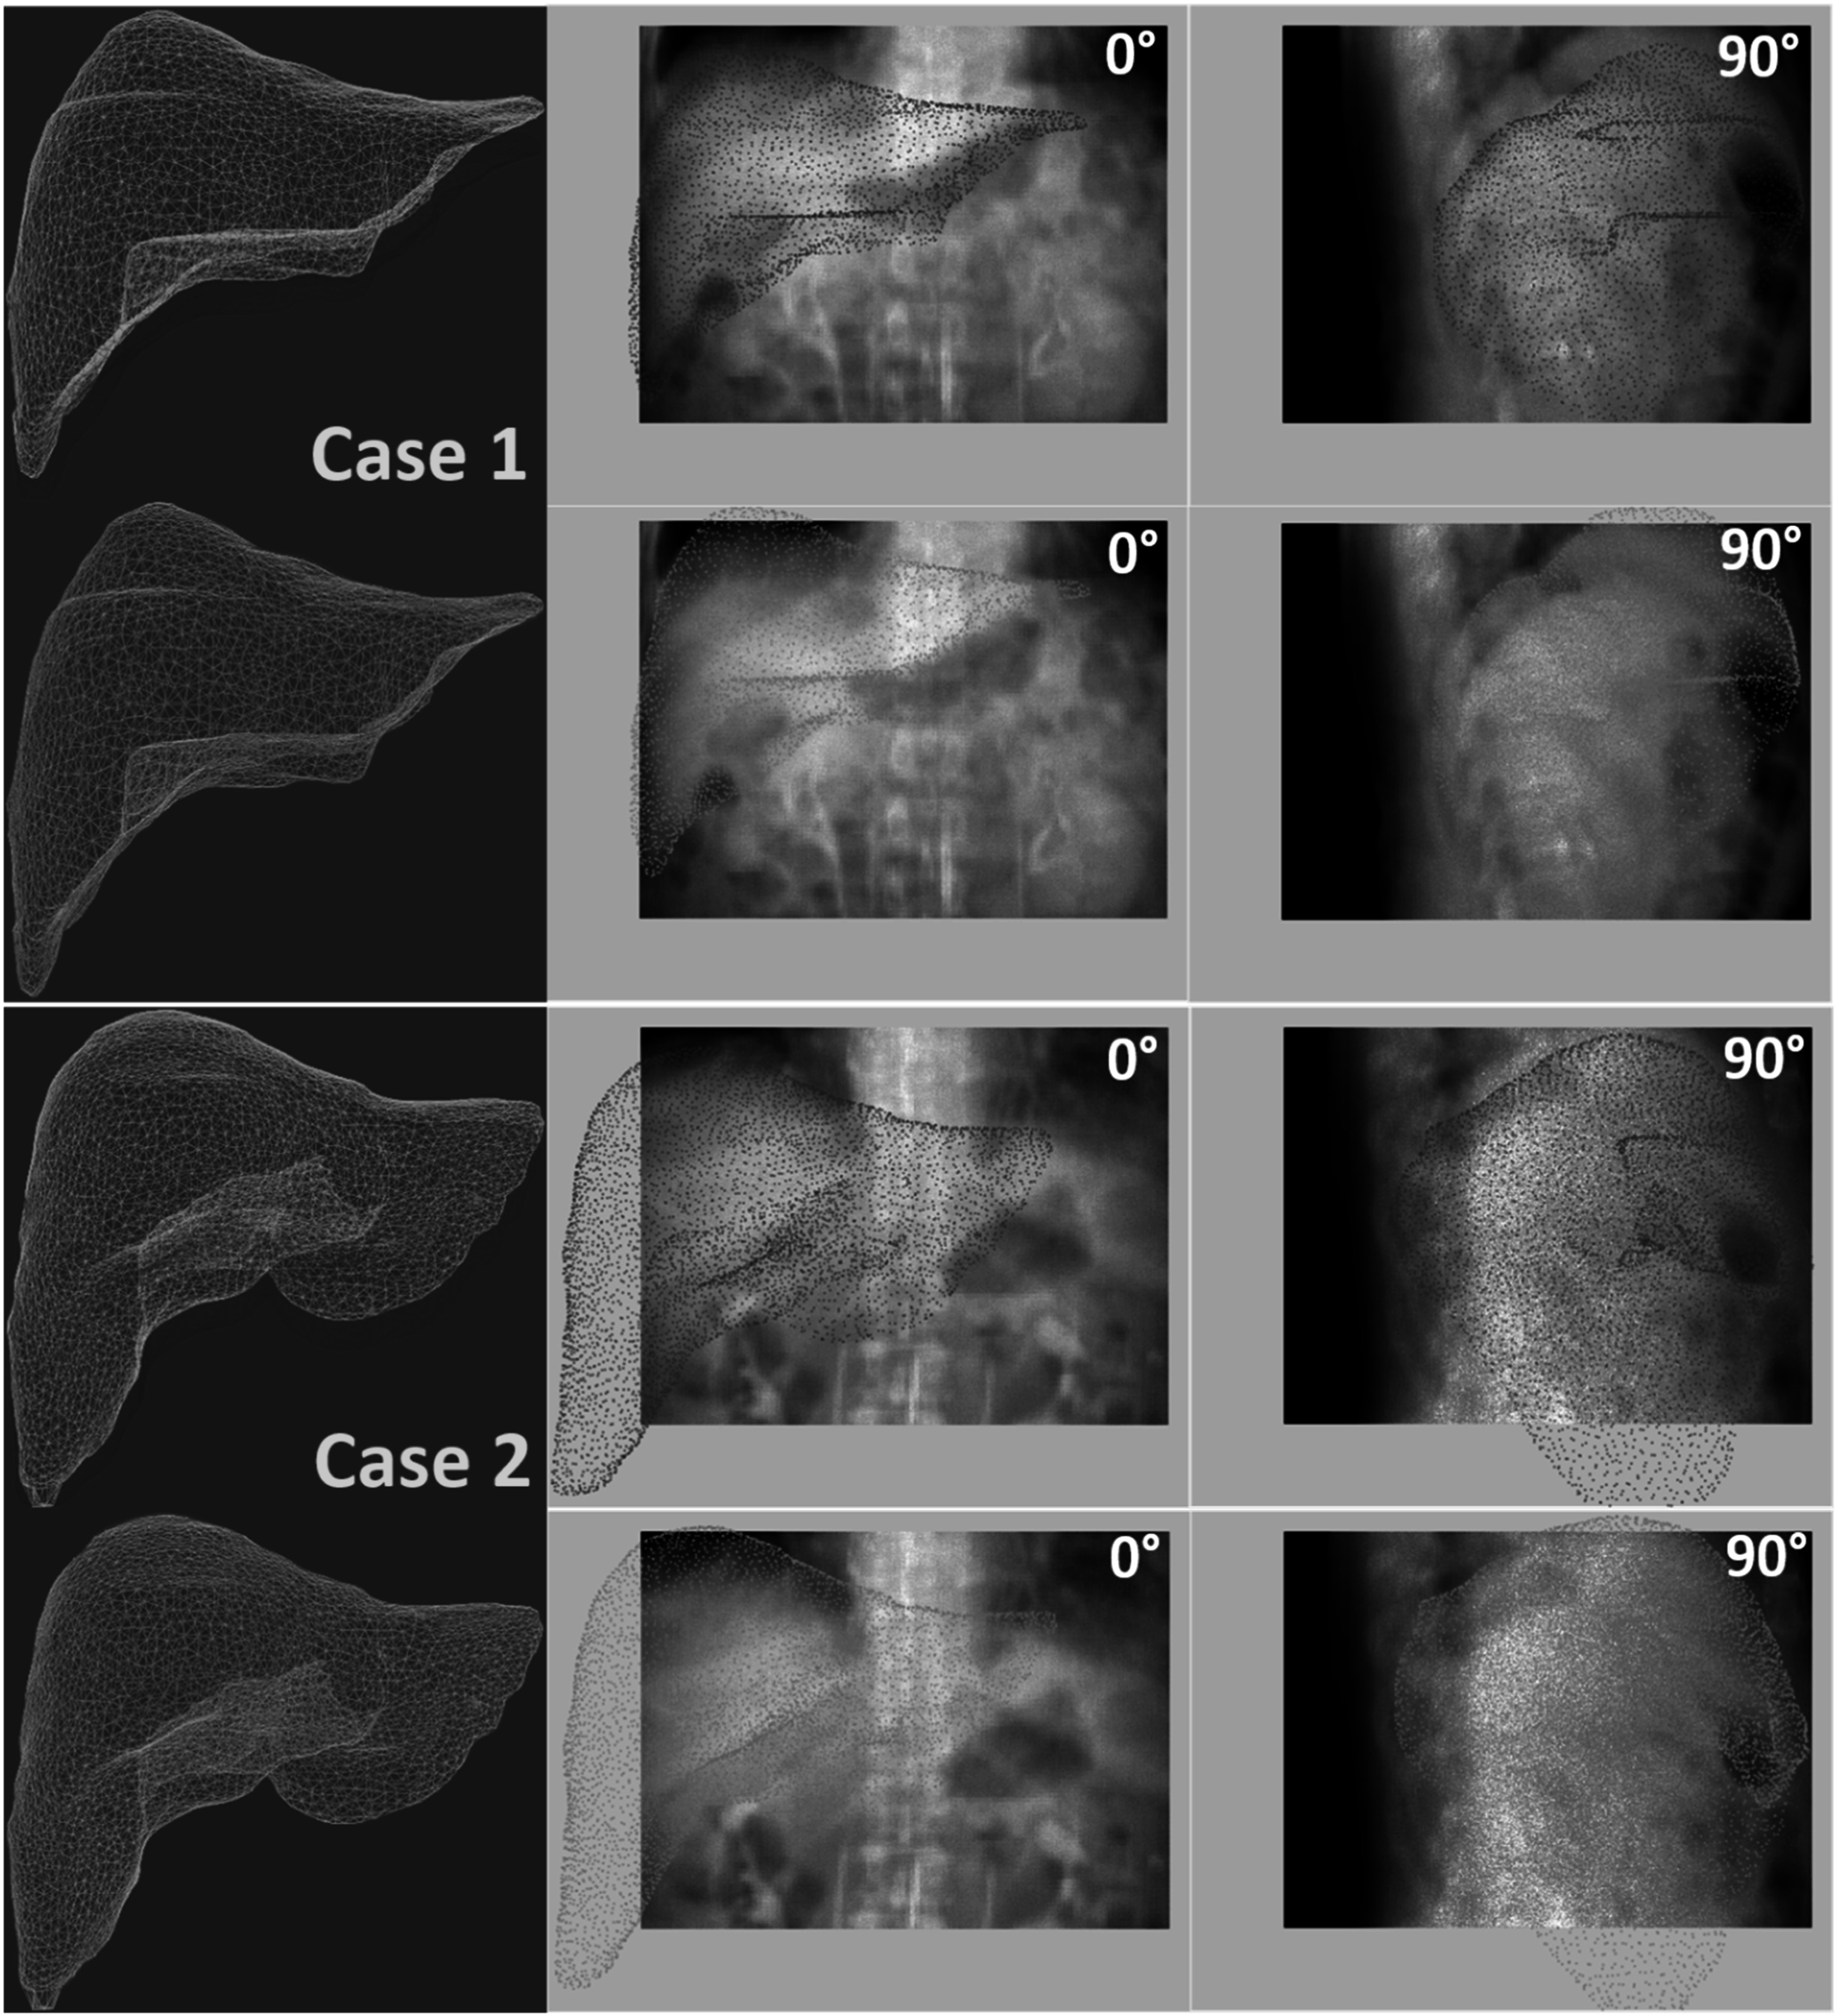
Liver boundary registration results of Surf-X-Bio for two patient cases. The 1st column shows the mesh overlays between the pre-registration (upper panel) and post-registration (lower panel) liver boundary meshes of each patient case. The ‘ground-truth’ target mesh is shown in yellow, and the prior and predicted meshes are shown in red and green, respectively. The other columns project the pre-registration and post-registration liver boundary nodes onto the x-ray projections at two projection angles (0° and 90°). The red and green dots represent the projected nodes of the prior and deformed liver boundary meshes, respectively.
